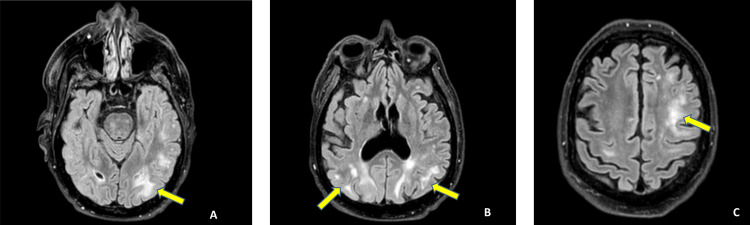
T2-FLAIR MRI of the brain showing features of PRES. Yellow arrows indicate areas of hyperintensity consistent with vasogenic edema in the parietal and occipital lobes. (A and B) PRES-associated changes in the occipital lobe. (C) PRES-associated changes in the parietal lobe. PRES, posterior reversible encephalopathy syndrome; FLAIR, fluid-attenuated inversion recovery
